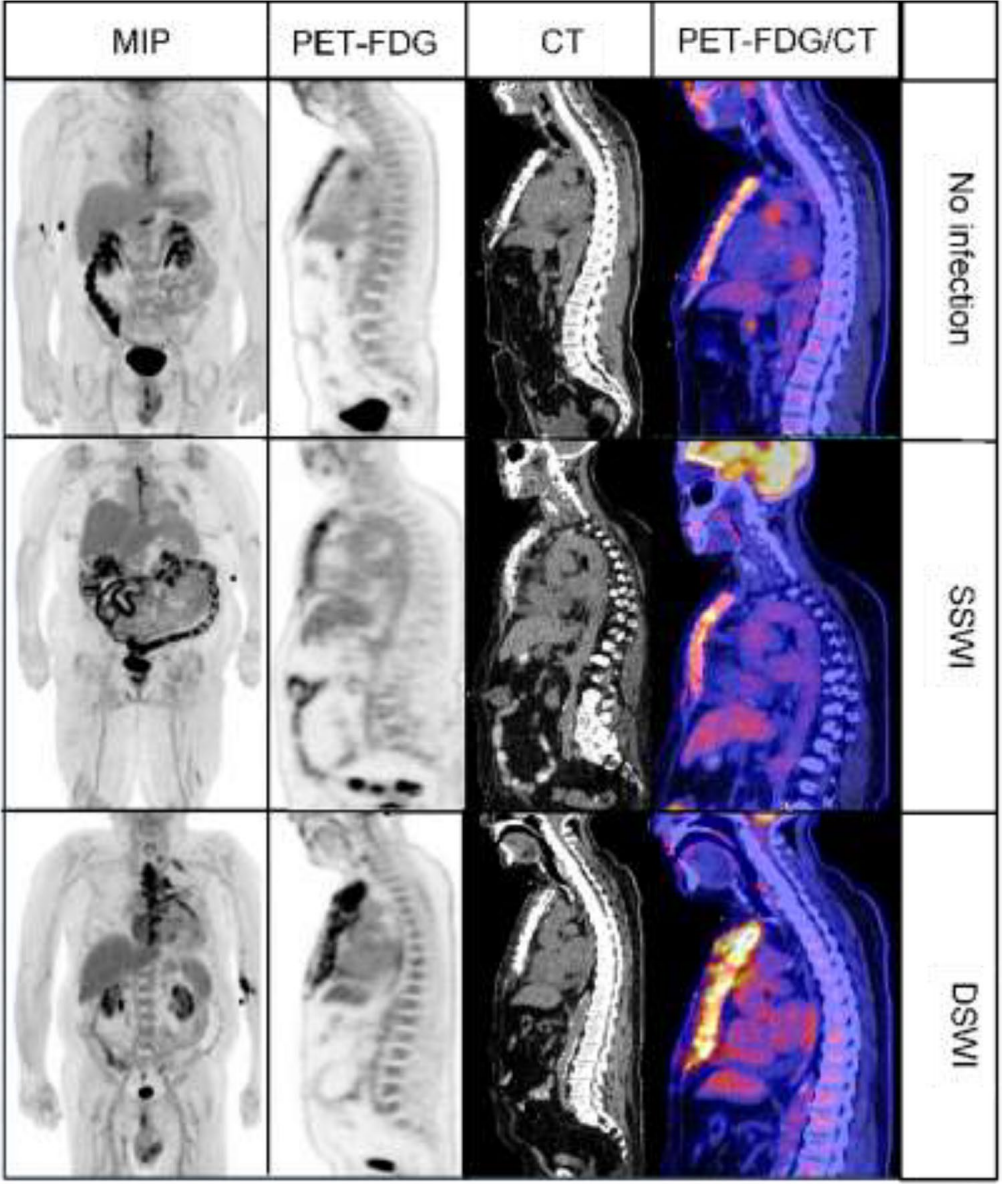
Anterior maximal intensity projection (MIP) images and sagittal PET, CT, and fused PET/CT images of the FDG sternotomy uptake patterns for no infection, superficial sternal wound infections (SSWI), and deep sternal wound infection (DSWI) referring to osteitis and/or mediastinitis
